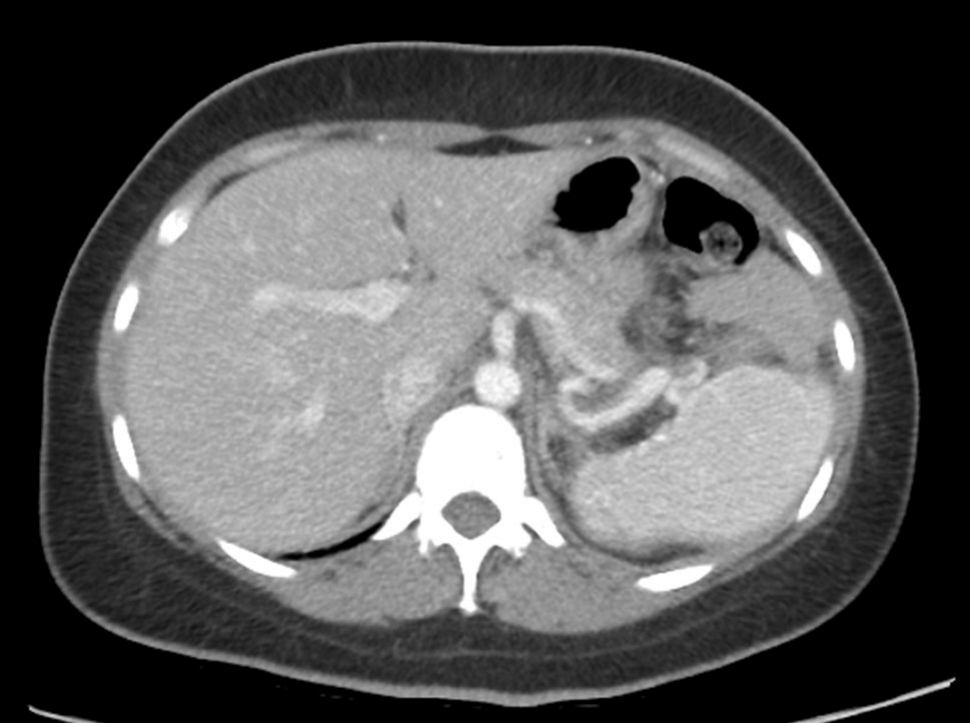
Post-operative CT scan demonstrating patent splenic vessels, homogenously enhanced spleen and no peri-splenic collections
